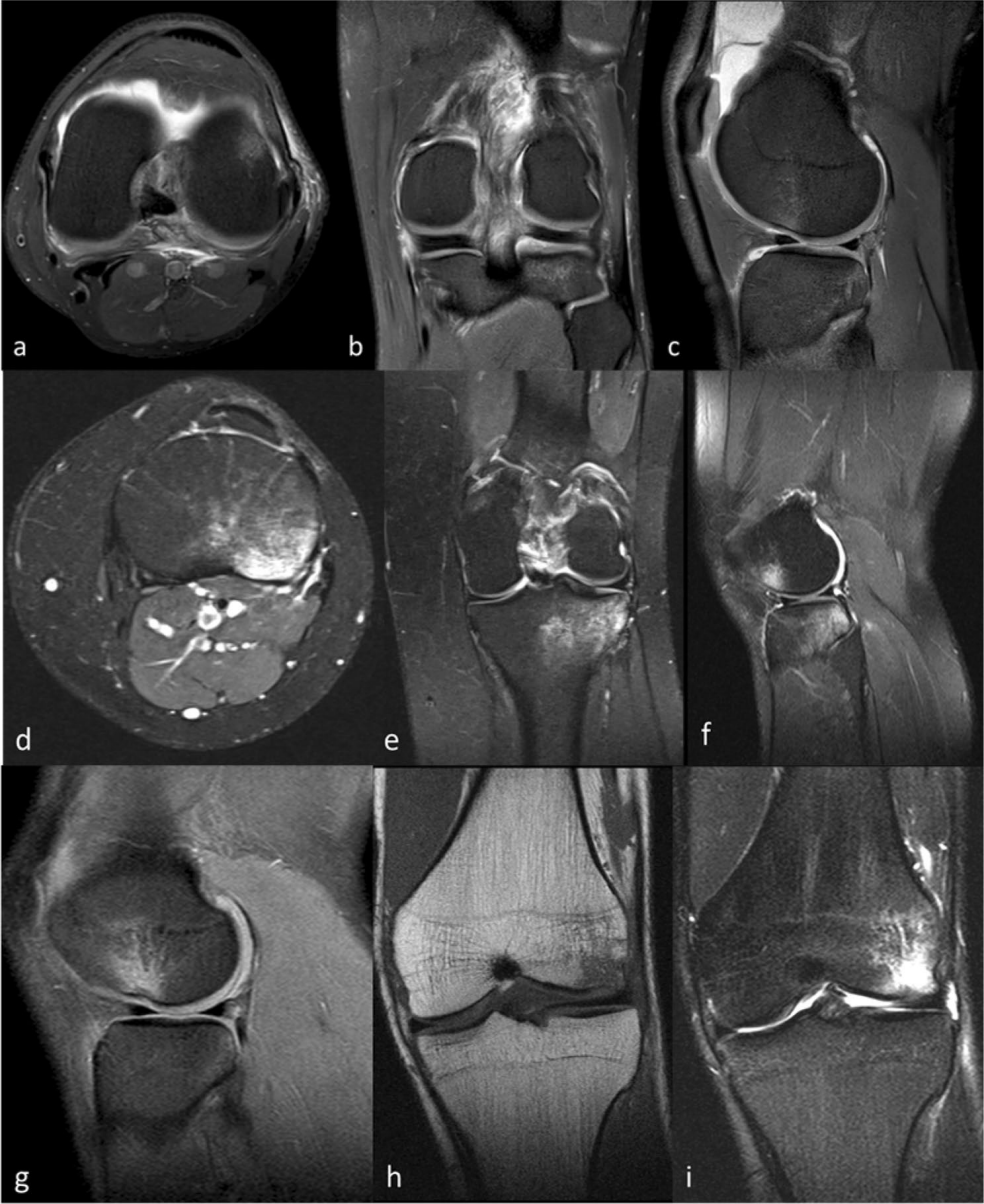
MRI examples of study patients presenting with posttraumatic BB accompanying ACL injury. a–c Costa-Paz type 1 of the lateral tibial plateau and lateral femoral condyle; axial, coronal and sagittal. d–f BB type Costa-Paz type 2 of the lateral tibial plateau and lateral femoral condyle; axial, coronal and sagittal. g–i Costa-Paz type 3 of the lateral femoral condyle with “lateral femoral notch sign”; sagittal and coronal. (a–g, i = PD tse, h = T1)
